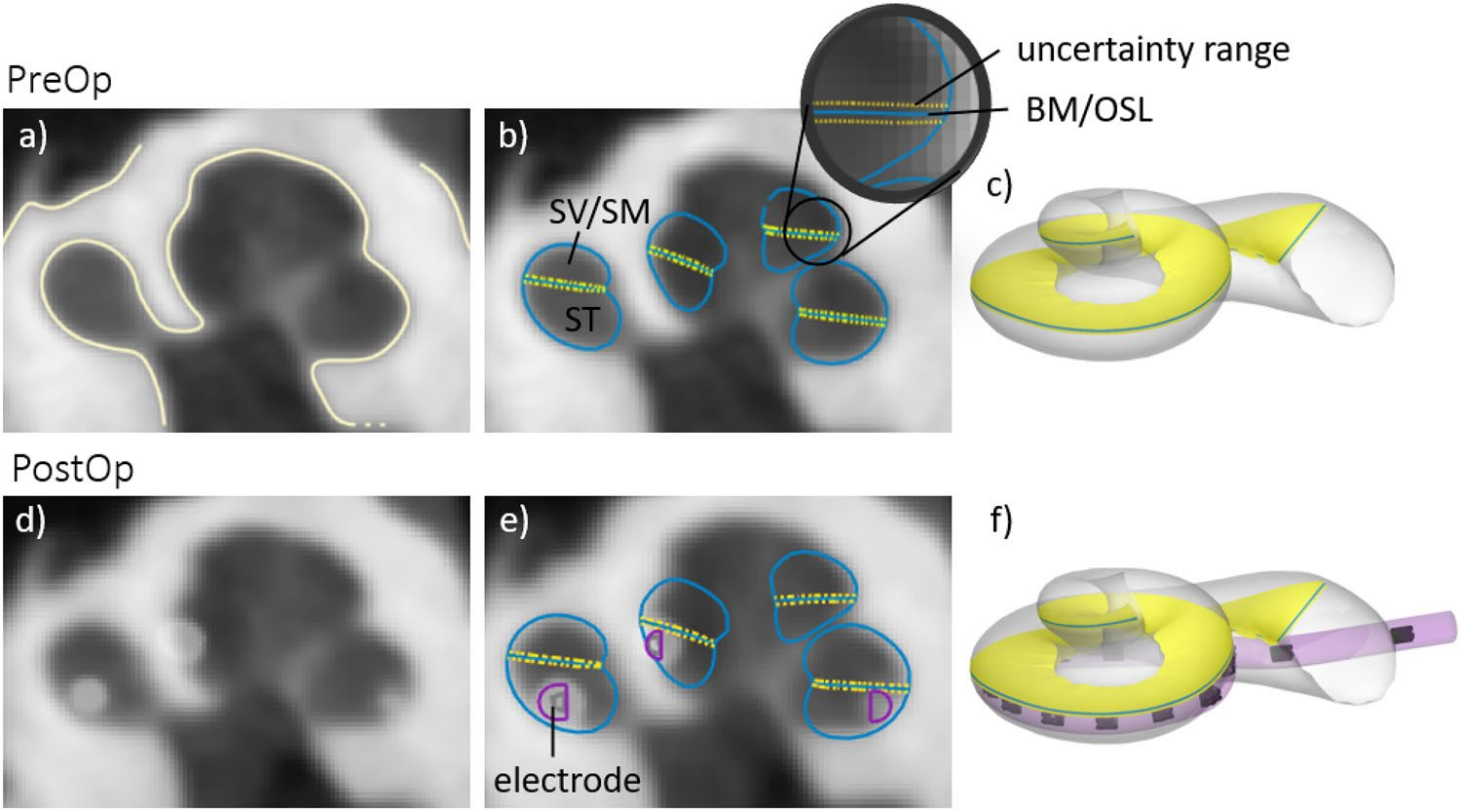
Image (a) shows the automatic segmentation of the cochlea. With a statistical shape model algorithm, a cochlear model (c) is fitted on the segmentation and superimposed on the CT scan (b). The model shows the scala tympani (ST), scala vestibuli and scala media (SV/SM) as well as the basilar membrane and osseous spiral lamina (BM/OSL) with the corresponding mean deviation as uncertainty range. Image (d) shows the postoperative CT scan registered on the preoperative CT scan. An algorithm detects the array contacts and generates a model of the electrode array (e,f).
